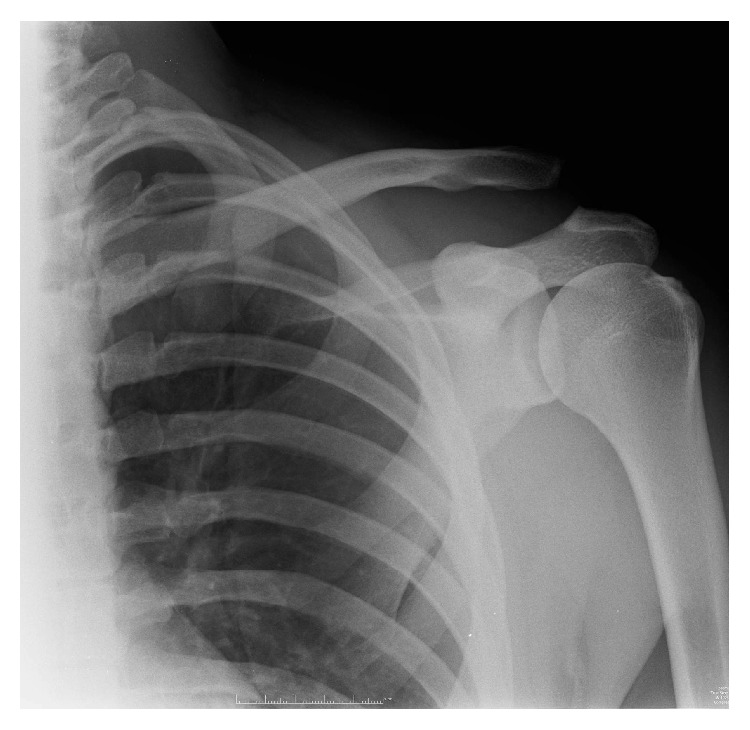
Anteroposterior left ACJ radiograph demonstrating Rockwood V ACJ dislocation.
